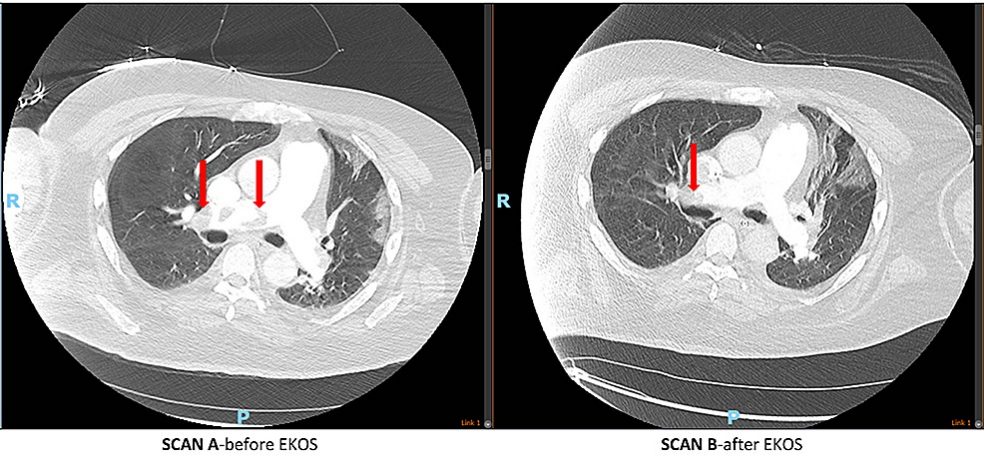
Minimal reduction in clot burden seen on CT chest pre and post EKOS procedure CT: computed tomography; EKOS: EkoSonic™ Endovascular System
